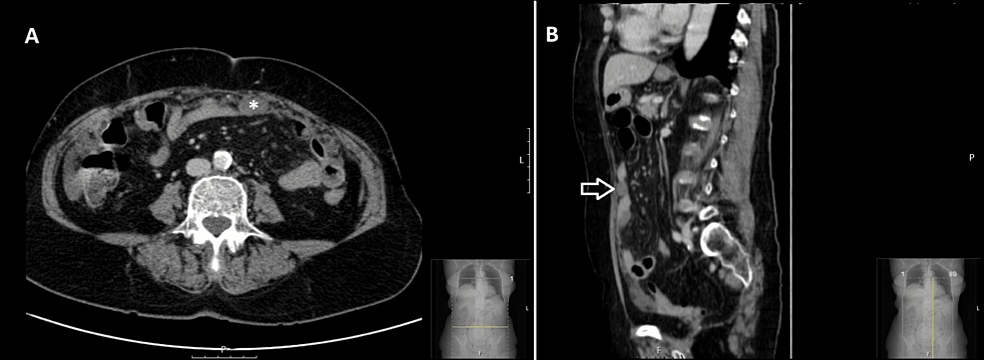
Axial (A) and sagittal (B) CT scan showing a peritoneal implant measuring 23 × 14 mm (asterisk in A and arrow in B), located in the periumbilical plane, slightly lateralized to the left CT: computed tomography
